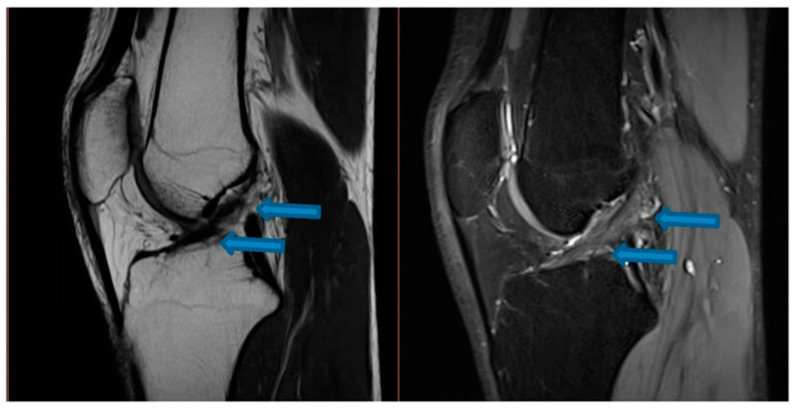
Sagittal T1- and T2-weighted MRI of the knee demonstrating signs of complete ACL healing indicated by blue arrows (14 August 2021).
